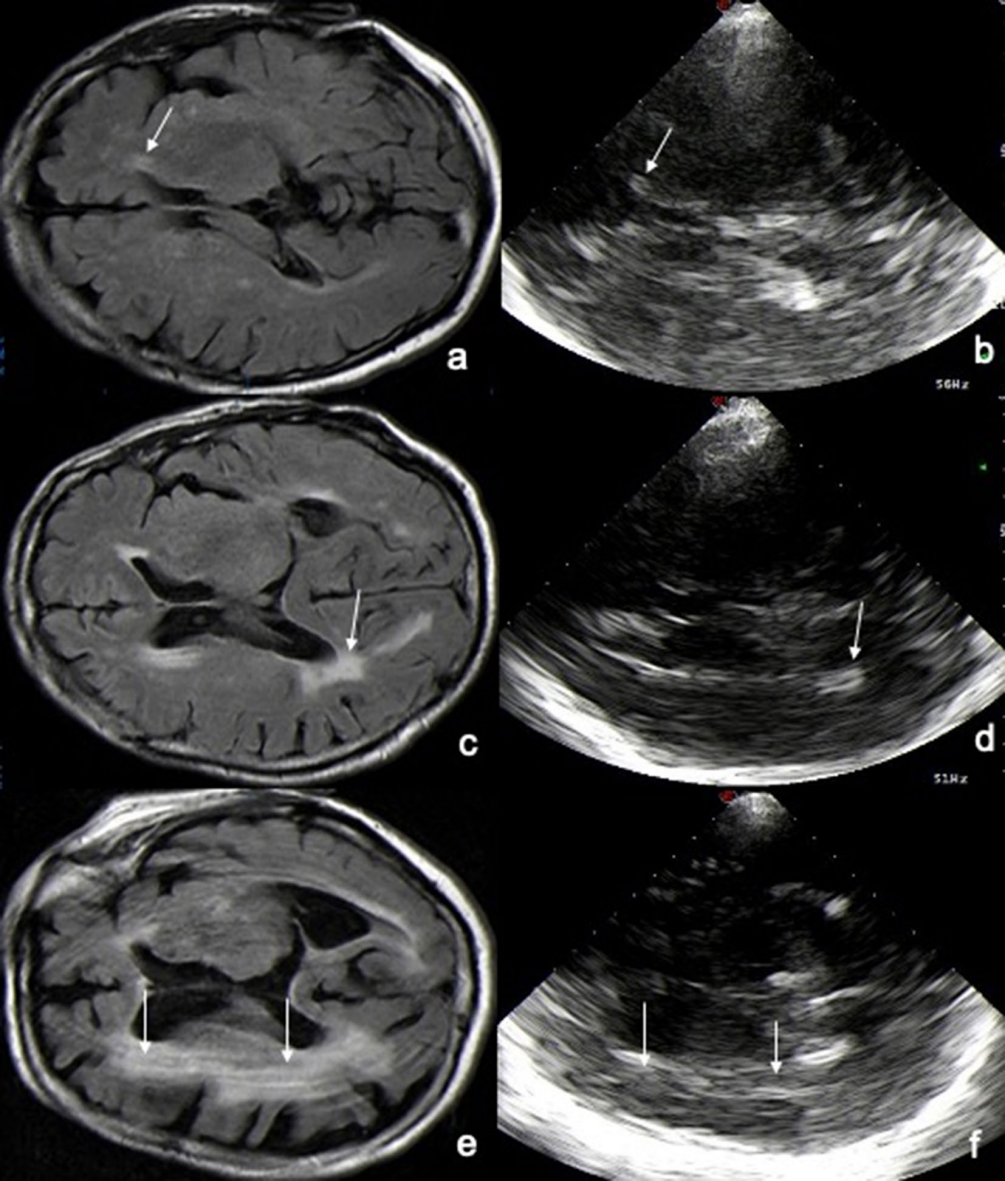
US visualization of WMLs based on MRI. The brain lesions seen on MR images correspond on ultrasound (US) images to hyperechogenic signals, double or blurred lines or as inhomogeneous hyperechogenic signal surrounding the wall of the central part, the frontal or dorsal horns of the lateral ventricles. Left: cerebral MR FLAIR image, axial plane. Right: transcranial sonography in B-Mode. a, b: WMLs I, white arrows indicate WML at the anterior horn ipsilateral. c, d WMLs II, white arrows indicate WML at the dorsal horn contralateral. e, f WMLs III, white arrows indicate WML in the deep white matter surrounding the central part contralateral
